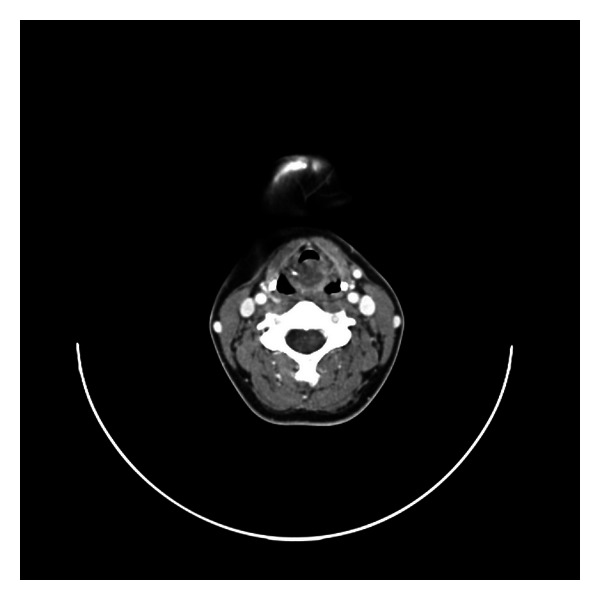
Serial CT axial images of the midline supraglottic mass commencing just below the aryepiglottic folds and extending onto arytenoids.
